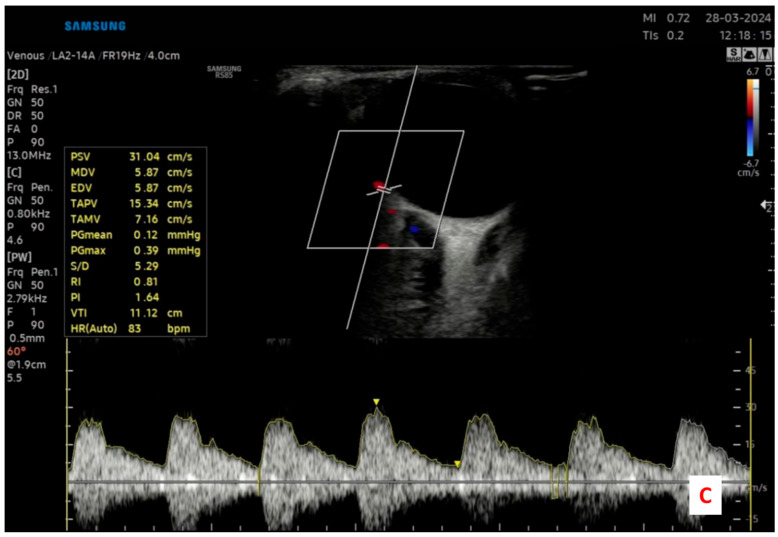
Representative color Doppler ultrasonography images demonstrating the retrobulbar blood flow assessment in a study participant. (A) Central retinal artery (CRA) identified within the optic nerve sheath; (B) Ophthalmic artery (OA) measured at the orbital apex; and (C) Posterior ciliary artery (PCA) evaluated near the optic nerve entry site.
